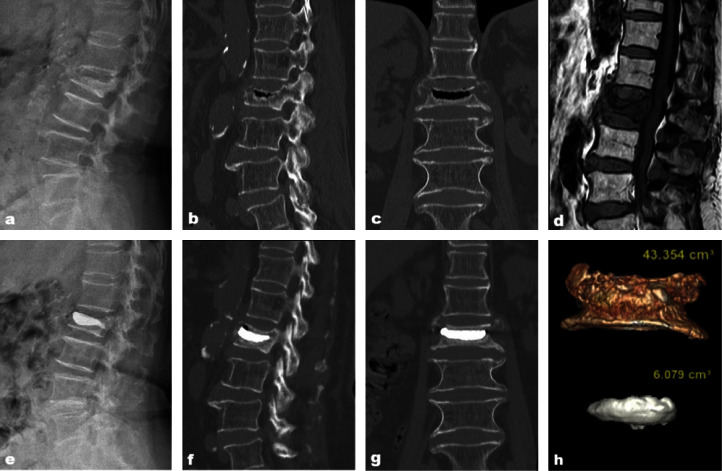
An 80-year-old female patient is diagnosed with an L2 osteoporotic vertebral compression fracture (OVCF). (a) Preoperative lateral X-ray. (b) Preoperative sagittal CT. (c) Preoperative coronal CT. (d) Preoperative MRI. (e) Postoperative lateral X-ray. (f) Postoperative sagittal CT. (g) Postoperative coronal CT. (h) 3D image of the vertebral body and bone cement.
